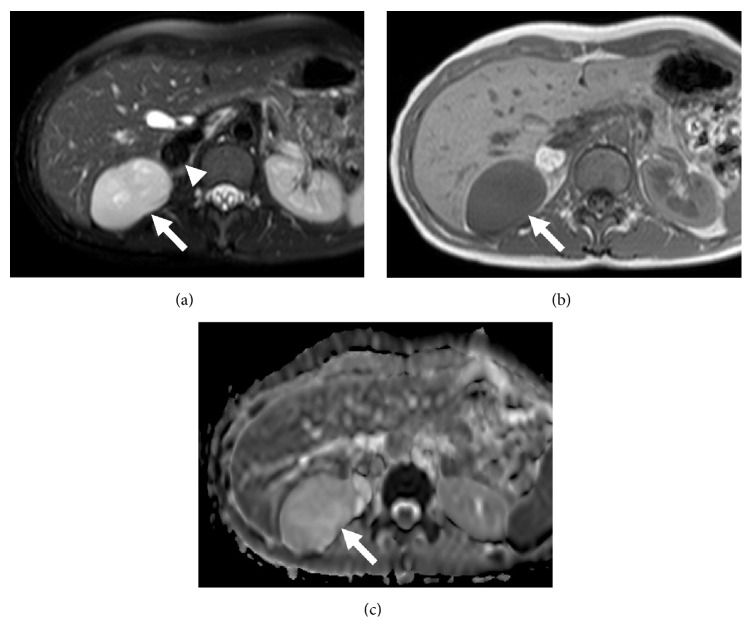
Axial MRI. (a) Fat-suppressed T2-weighted image showed heterogeneous high intensity inside the cystic component of the mass (arrow). The fatty component showed low intensity (arrowhead). (b) T1-weighted image showed relative homogeneous isointensity inside the cystic component of the mass (arrow). (c) ADC map showed slightly restricted diffusion compared with cerebrospinal fluid. Its ADC value was 2.0 × 10^−3^ mm^2^/sec.
